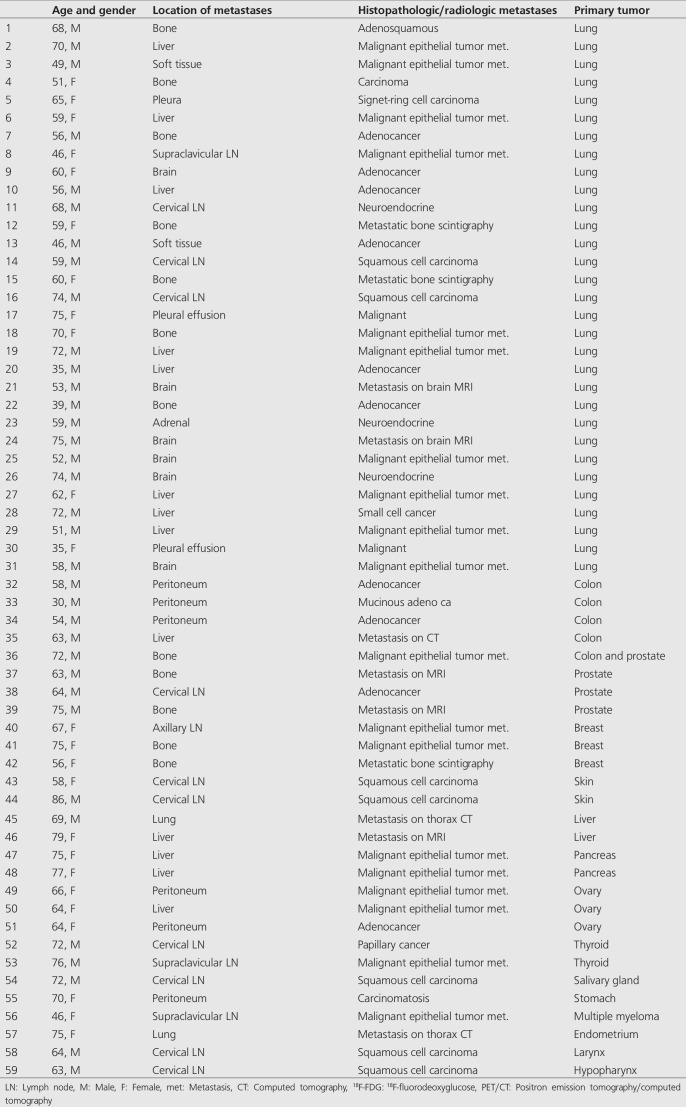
There were fifty-nine patients with sixty true-positive results diagnosed by ^18^F-fluorodeoxyglucose positron emission tomography/computed tomography
